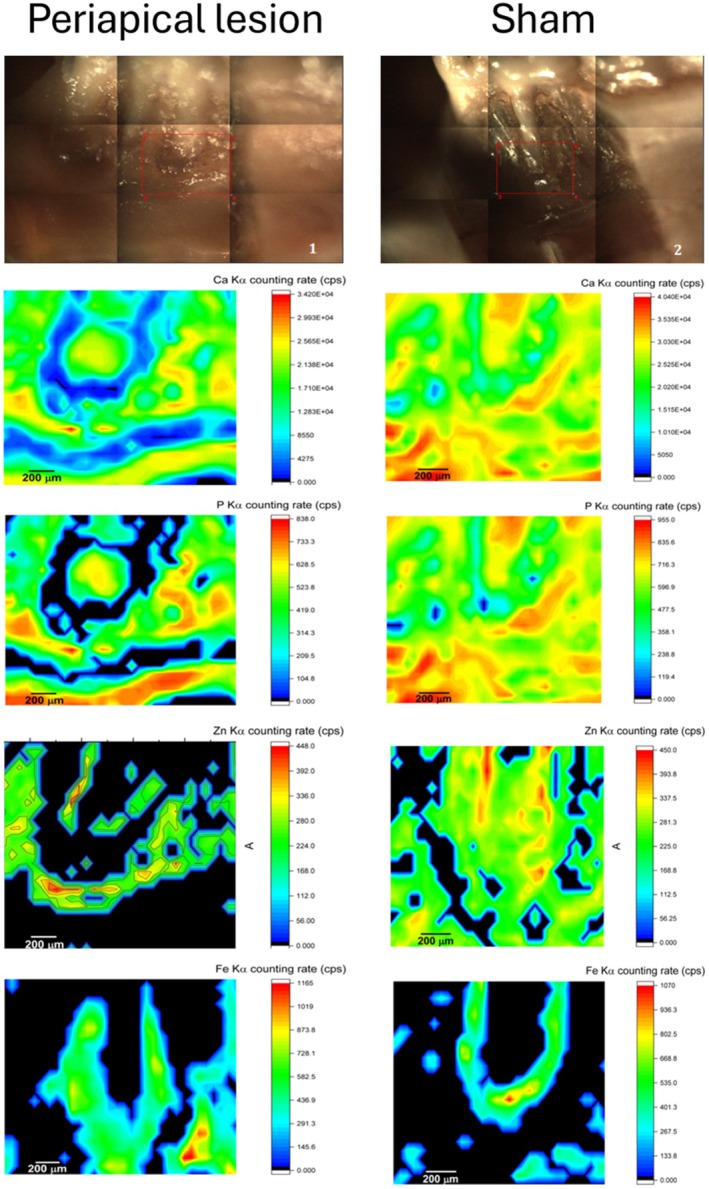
Comparative analysis between the periapical lesion and control (sham) conditions using μ‐XRF elemental distribution maps. Optical image of the analysed area in both conditions (1—Periapical Lesion and 2—Sham). The calcium (Ca Kα) map demonstrates a reduction in calcium intensity in the induced periapical lesions, indicating mineral loss, in contrast to the more homogeneous distribution in the sham controls. The phosphorus (P Kα) map corroborates this lower concentration in the periapical lesion, reflecting demineralization, whilst the concentration is maintained in the sham controls. The zinc (Zn Kα) map shows a more irregular distribution in the periapical lesion, whilst the sham controls exhibit a more uniform distribution. Finally, the iron (Fe Kα) map displays higher signal intensity in the induced periapical lesions, whilst in the sham controls, its levels are less intense.
